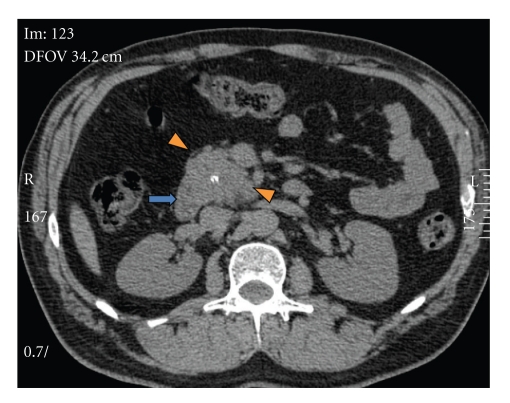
Axial noncontrast CT abdomen demonstrates a retroperitoneal soft tissue lesion (arrowheads) with lobulated margins and a central calcification. Prominent vessels are noted around the lesion. The second duodenal portion contacts the mass (arrow).
